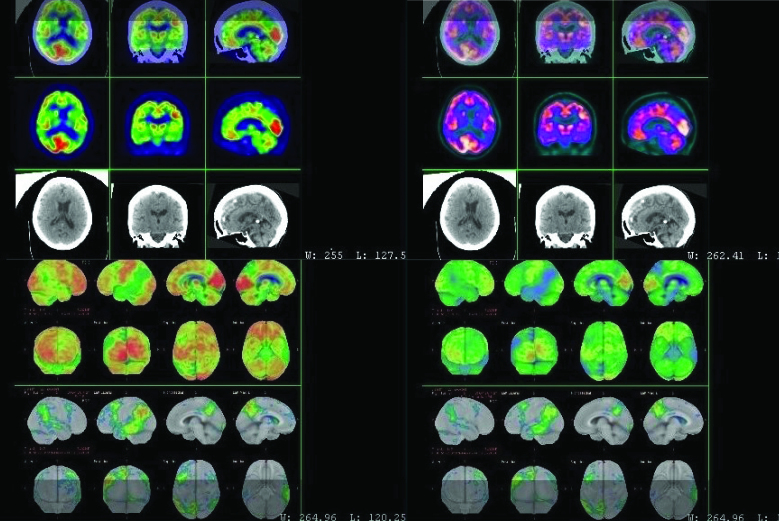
Fluorodeoxyglucose-positron emission tomography (FDG-PET) sequences showing moderate/marked reduction in glycolytic metabolism in the lateral regions of the left temporal lobes and the lower portions of the parietal lobes.
